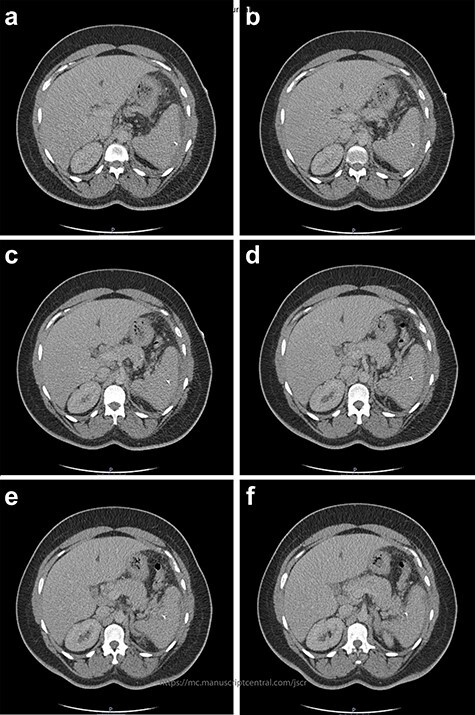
Two-mm axial sections from a CT scan demonstrating the linear hyperdensity traversing the spleen across multiple cross sections. (a) Section number 65 (b) section number 67 (c) section number 69 (d) section number 71 (e) section number 73 (f) section number 75.
